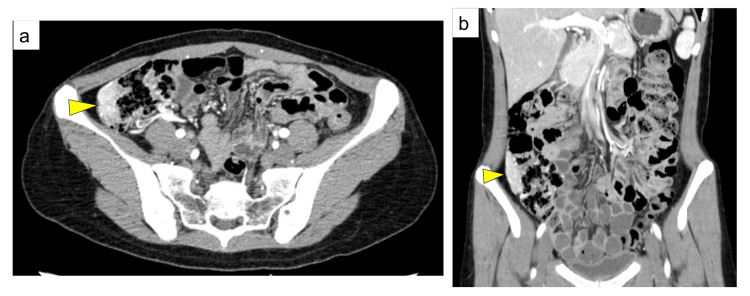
Computed tomography (a) Transverse computed tomography shows a tumor circumferentially involving the right wall of the ascending colon (arrowhead). (b) Coronal computed tomography reveals a 40-mm tumor extending along the craniocaudal axis in the ascending colon (arrowhead), with early contrast enhancement suggesting hypervascularity.
